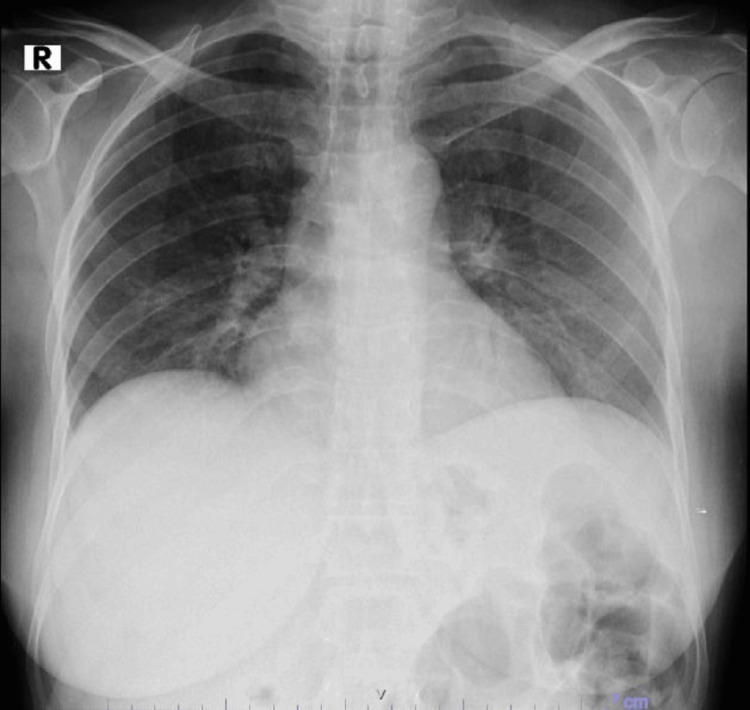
Admission chest X-ray (normal)
